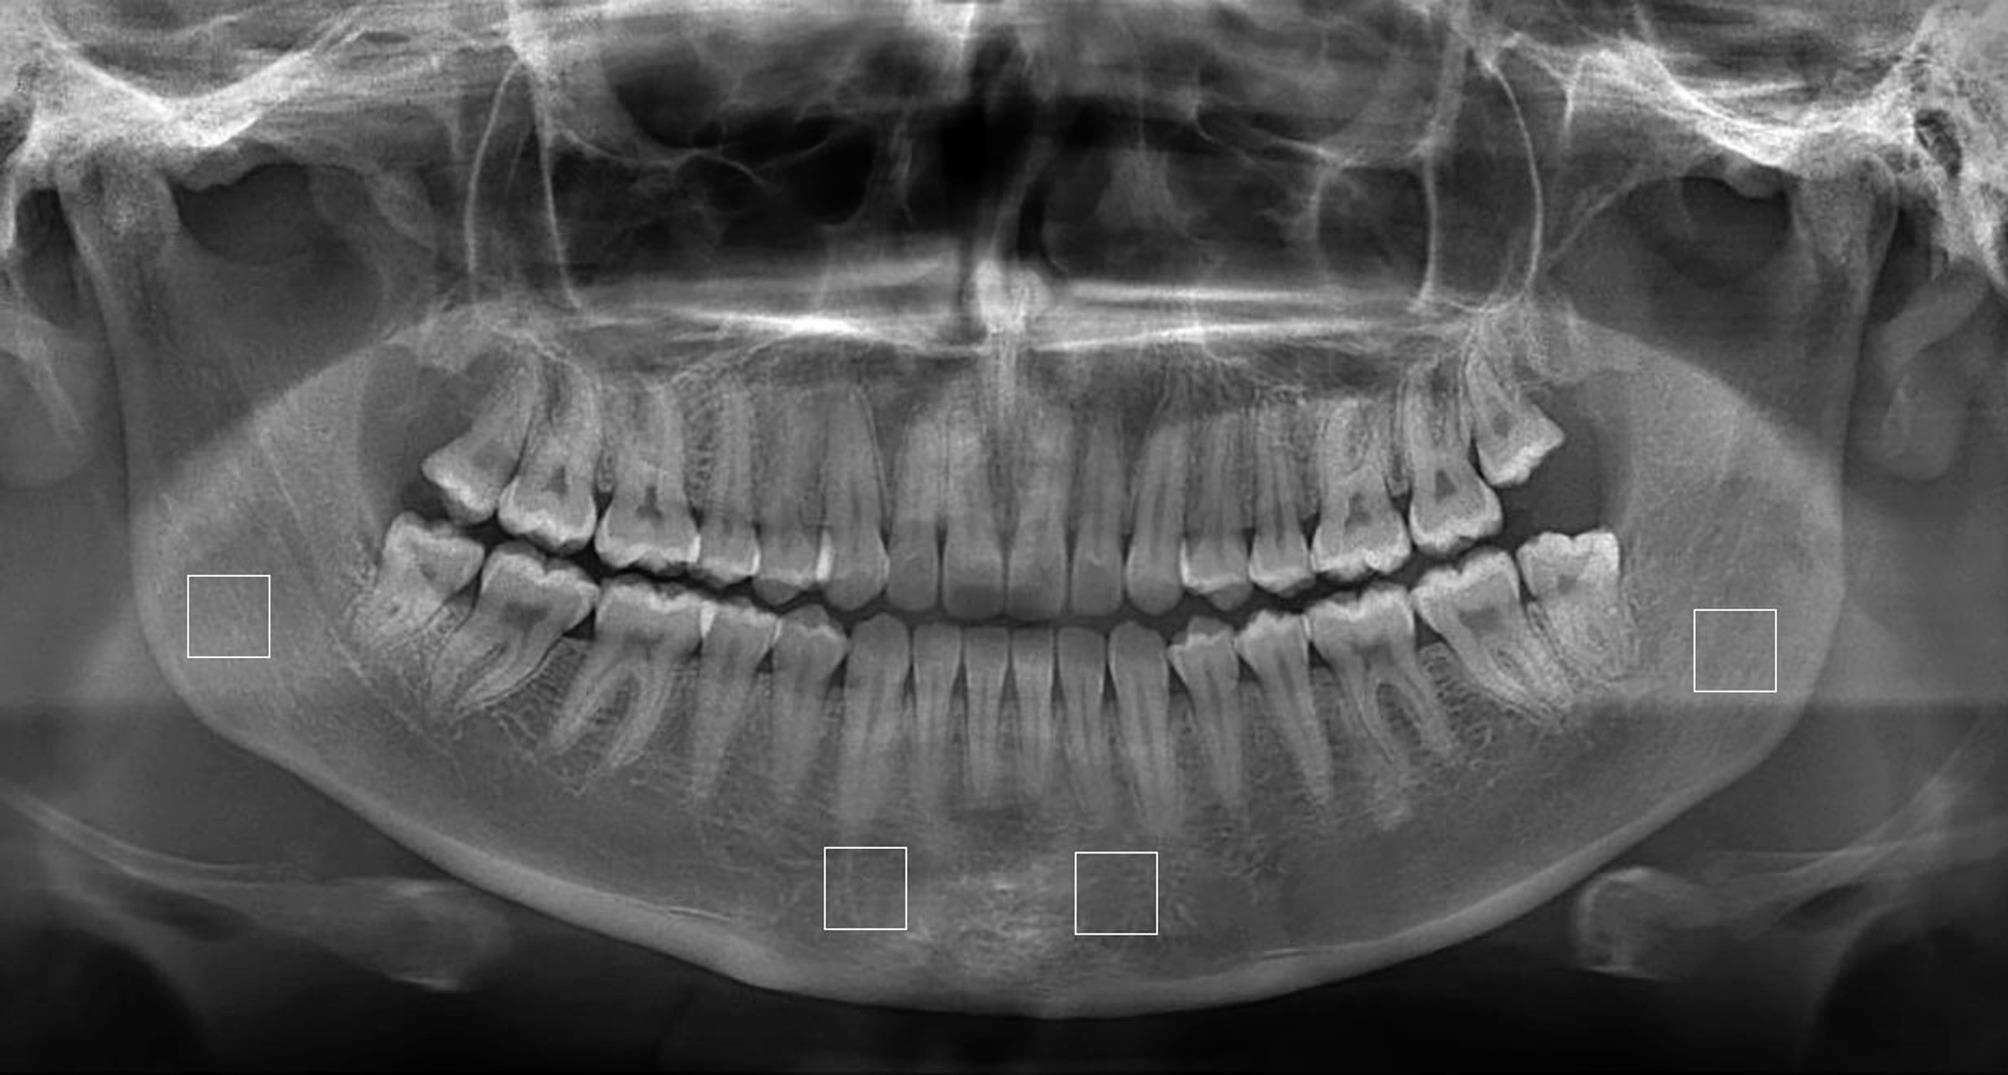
Obtaining ROIs on a panoramic radiograph
